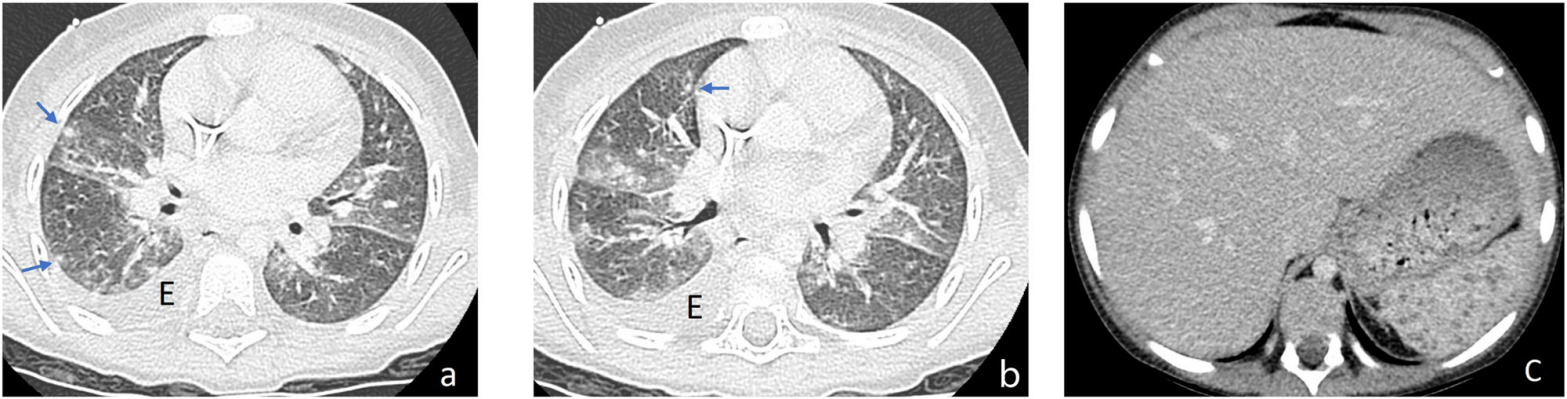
Proven invasive candidiasis in a 4-year-old boy with pre-B cell acute lymphoblastic leukemia (ALL) under chemotherapy. The patient developed non-productive cough, fever, and skin nodules in agranulocytosis. Axial chest contrast-enhanced computed tomography (CE-CT) images in lung window (a, b) show multifocal areas of ground-glass infiltrates, multiple small-sized lung nodules (blue arrows) and moderate right pleural effusion (E). Although the findings were nonspecific, the development of synchronic skin nodules and splenic lesions (c) highly suggested disseminated candidiasis, confirmed by biopsies of the cutaneous nodules and buccal mucosa
